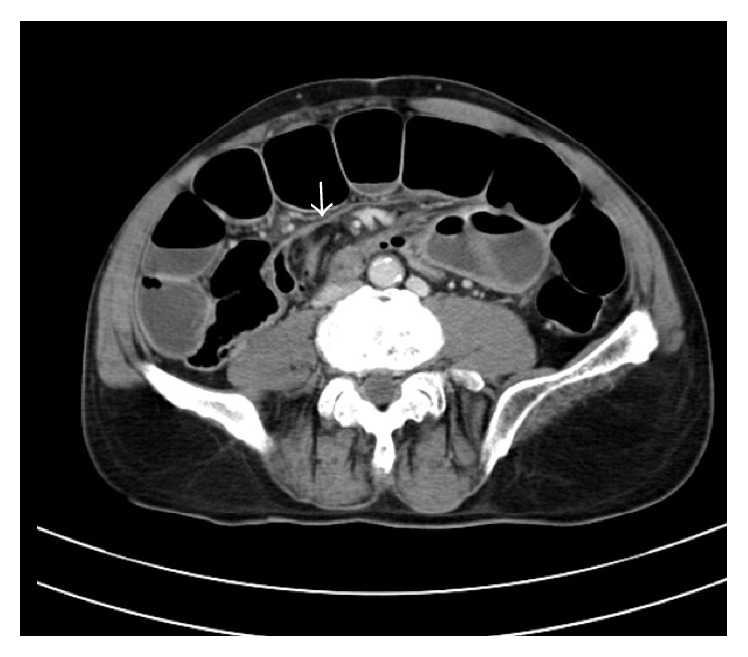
CT abdomen axial view showing band like structure extending from the distal ileum (white arrow).
